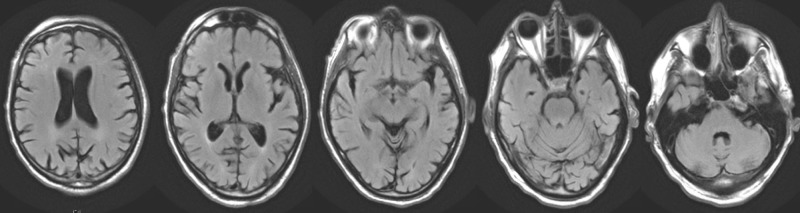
T2 Flair Propeller T2 flair propeller magnetic resonance imaging (MRI) showing a regular brain with no evidence of pathology.
